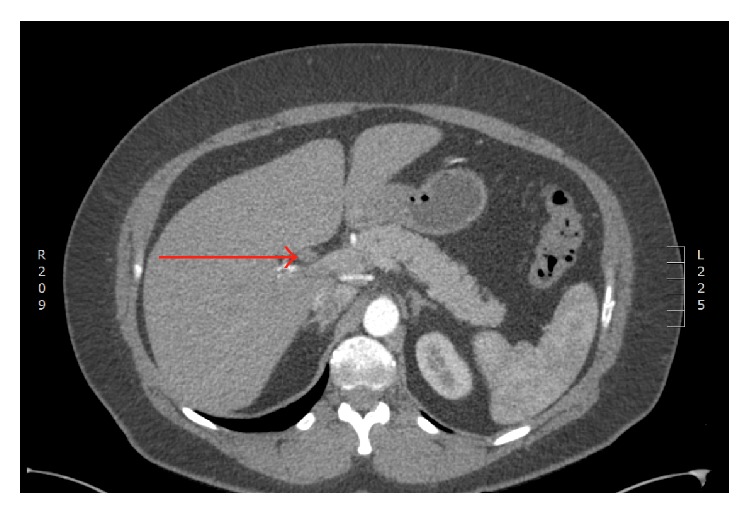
Contrast-enhanced computed tomography (CT) scan demonstrating an arterially enhancing filling defect within the proximal hepatic duct (marked with red arrow).
